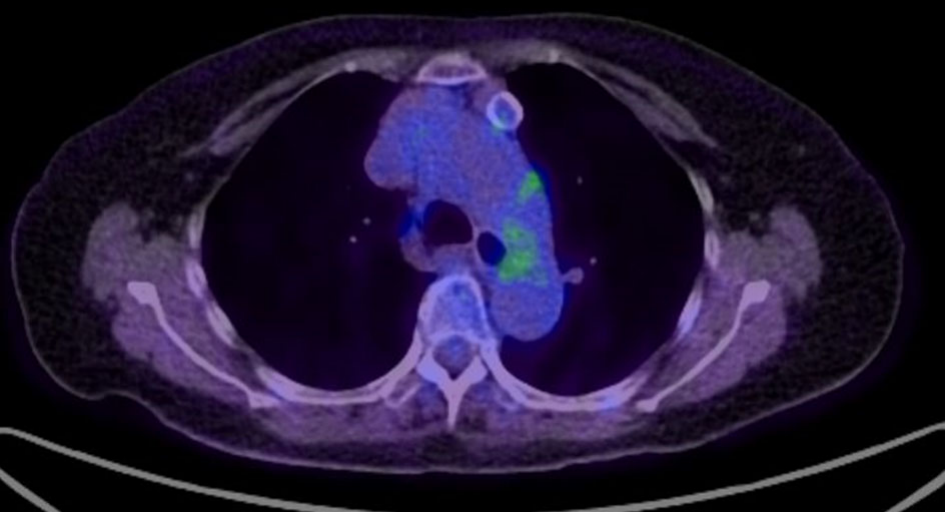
F^18^ fluorodeoxyglucose‐positron emission tomography (F^18^ FDG‐PET) showed no uptake of FDG in the anterior mediastinal tumor
